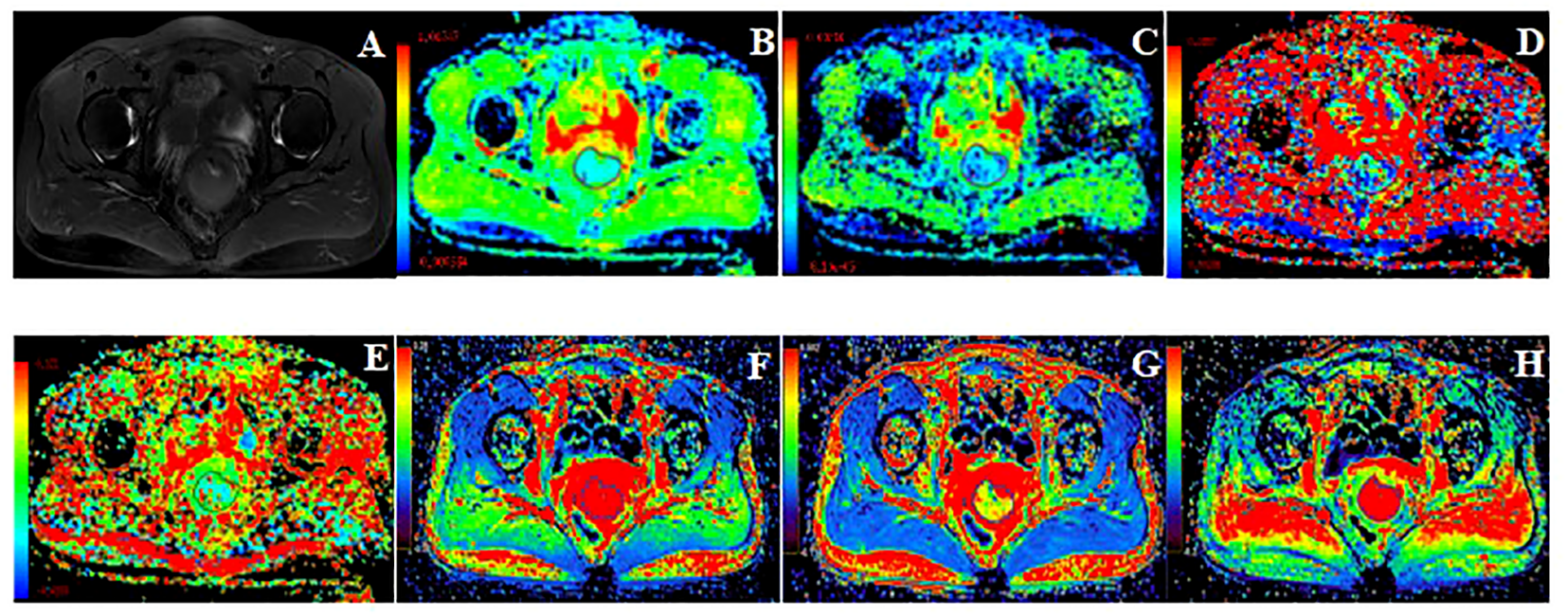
49 years old, FIGO IB3 cervical cancer with PLNM. (A): Axial T2WI image, (B–H): Pseudo-color images of each quantitative parameter of DCE-MRI and IVIM-DWI, (B): ADC_min_ was 0.47×10^-3^mm^2^/s, ADC_mean_ was 0.61×10^-3^mm^2^/s, ADC_max_ was 0.85×10^-3^mm^2^/s (C): D was 0.49×10^-3^mm^2^/s, (D): D* was 30.50×10^-3^mm^2^/s, (E): f was 0.12, (F): Ktrans was 0.49 min^-1^, (G): V_e_ was 0.27, (H): K_ep_ was 1.79 min^-1^.
